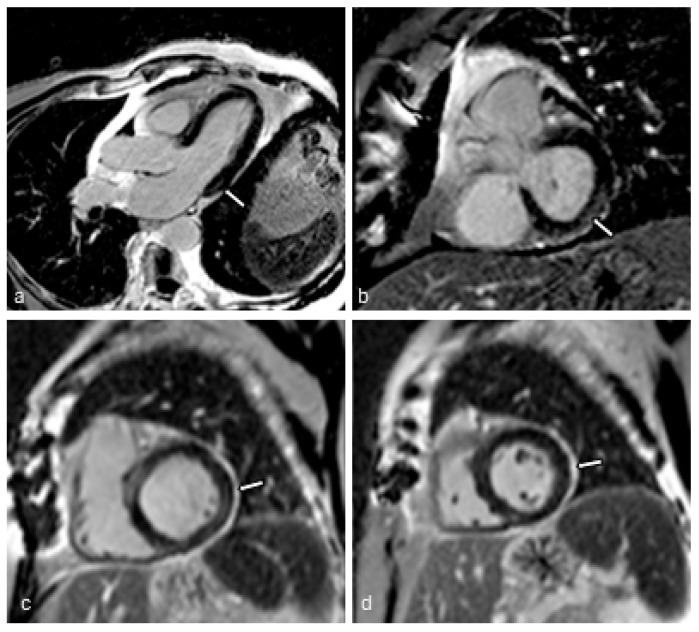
Cardiac MRI images from two COVID-19 survivors. Inversion recovery late gadolinium enhancement (LGE) images in three-chamber (a) and short axis (b) views of a 71-year-old man without hypertension, depicting mild non-ischemic myocardial LGE at the inferolateral wall, with an end-diastolic volume index (EDVI) of 59 mL/m^2^ and a 62% ejection fraction (EF). (c,d) Inversion recovery LGE images in short axis views of an 85-year-old woman with hypertension showing pericardial LGE, with an EDVI of 74 mL/m^2^ and a 61% EF.
